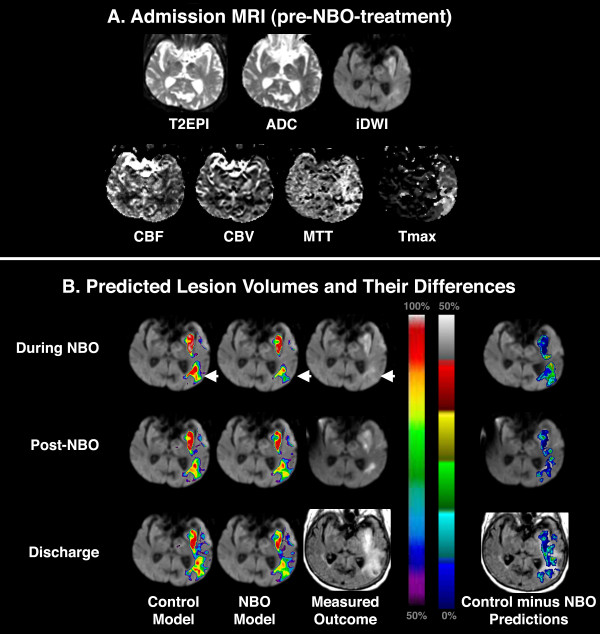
Example 1 of Predicted Lesion Volume Development. (A) Admission MRI dataset for a 79 year-old woman with stroke who was imaged 13 hours after she was last seen well and treated with NBO. (B) Corresponding GLM-predicted lesion risk maps (left panel) for Control and NBO-models at each subsequent time point of imaging, and overlay map (right panel) of differences between the two models showing ischemic tissue that is 'potentially salvageable' with NBO therapy. The GLM-predicted lesion volumes are asynthesis of data from the admission MRI only. In this patient, the risk of tissue infarction in DWI/PWI mismatch regions was predicted to increase over time. For clarity, only GLM-predicted lesion risk > 50% are shown overlaid on acute DWI. Note that the amounts of tissue predicted to infarct at all time-points with the Control-models were greater than their NBO-model counterparts, with difference principally in the DWI/PWI mismatch region (arrowheads). In the difference maps (B, right panel), the color scale represents infarction risk reduction as a result of NBO-therapy (conversely, larger values represent greater likelihood of infarction if the patient was given Control-treatment).
